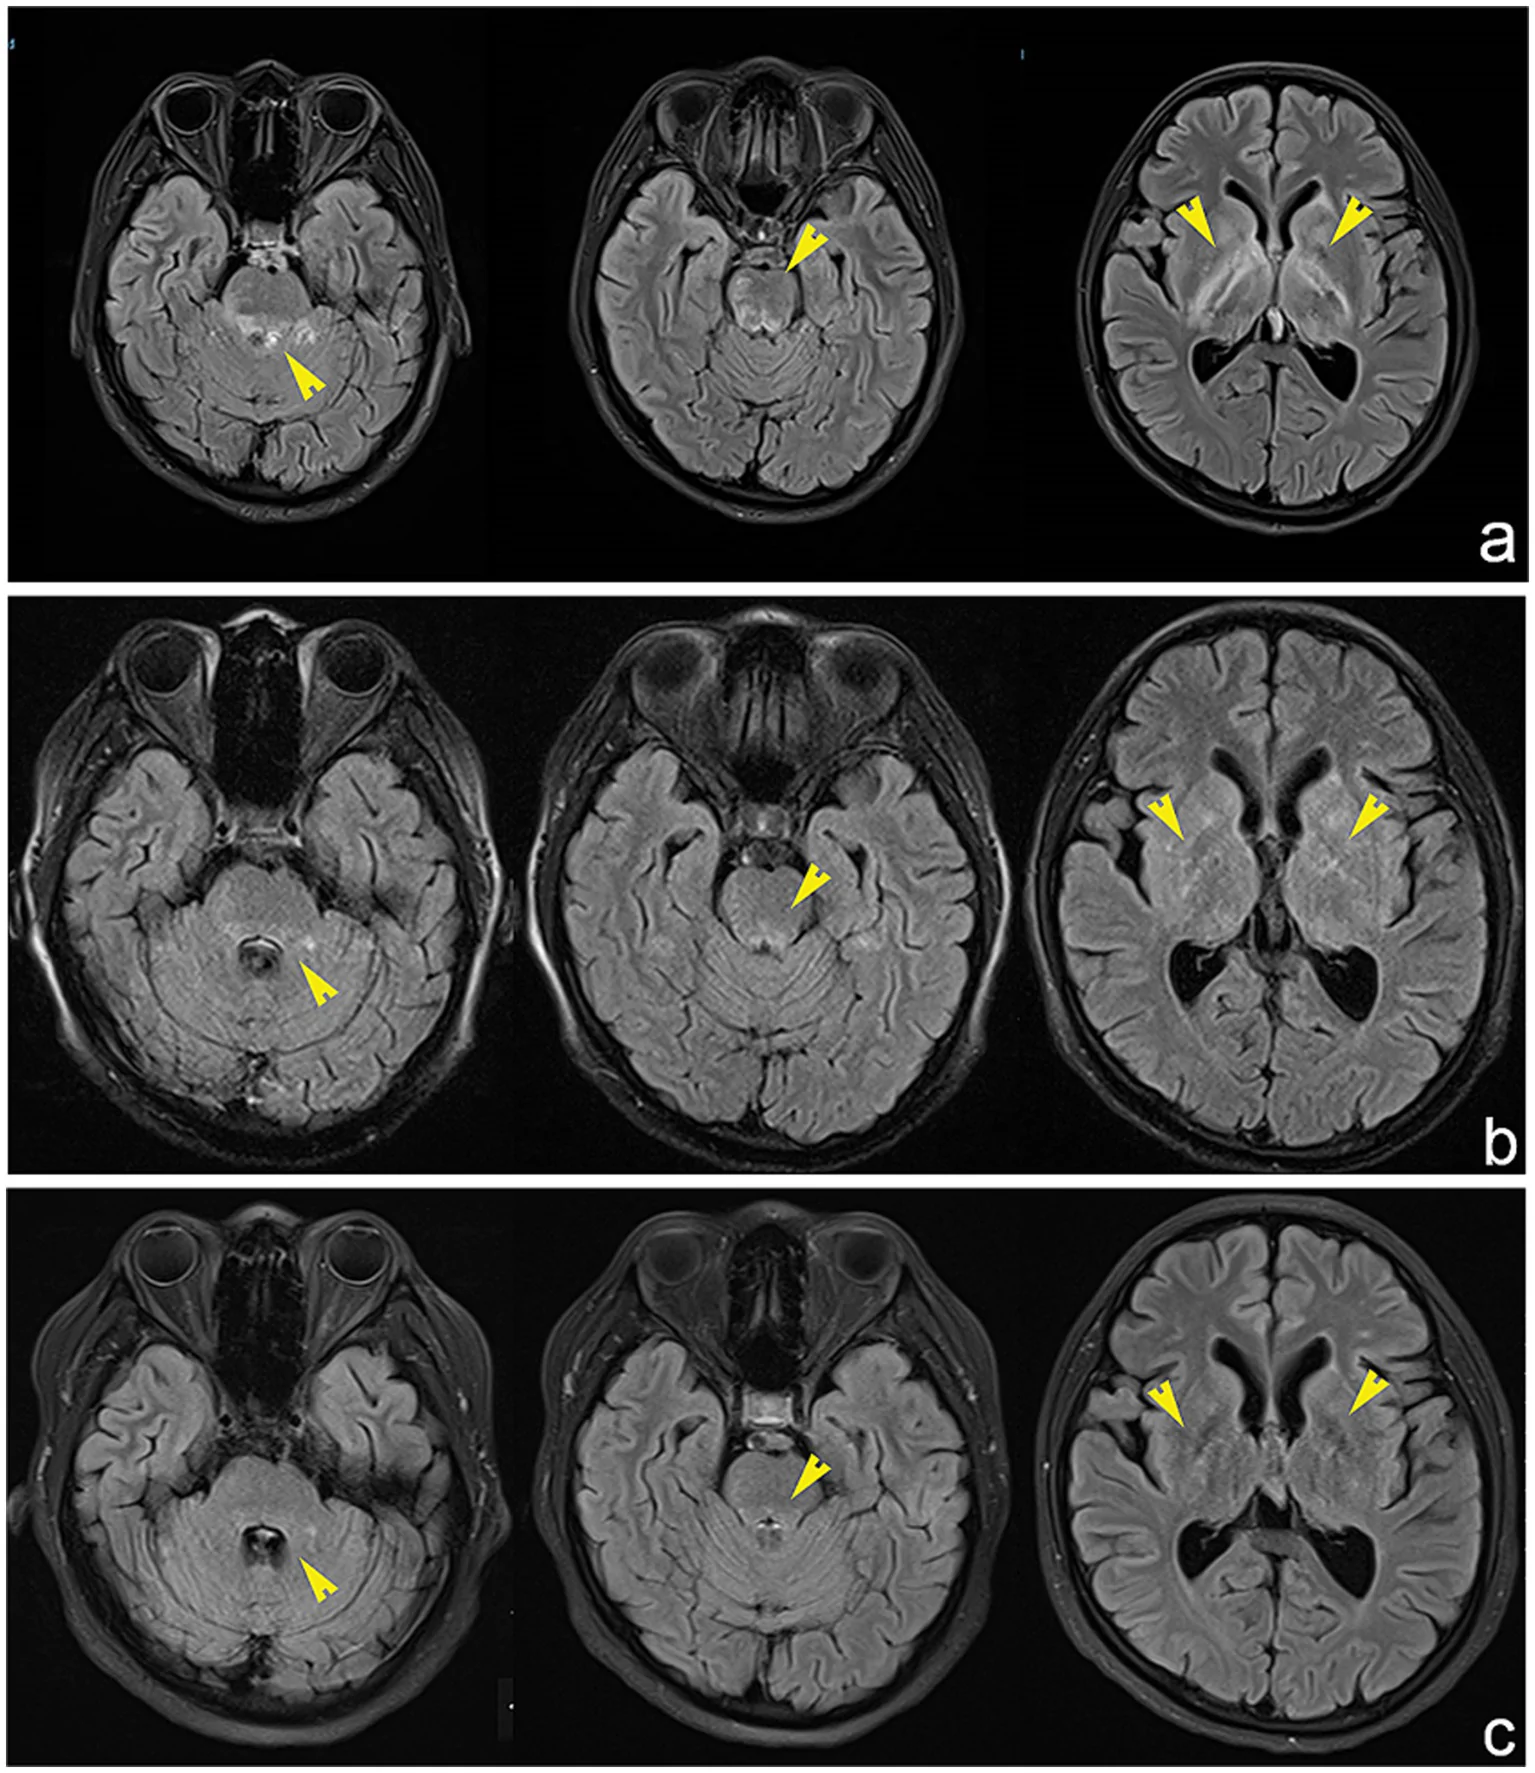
MRI showed marked reduction or complete resolution of the abnormal signals at one- and three-month post-treatment. (a) Before treatment, FLAIR images revealed multiple hyperintense lesions (yellow arrows) involving the pons, bilateral middle cerebellar peduncles, thalami, and basal ganglia. (b) At 1 month post-treatment, the lesions (yellow arrows) had dramatically shrunk. (c) At 3 months post-treatment, FLAIR sequences revealed that the original abnormal signals (yellow arrows) were largely obliterated.
